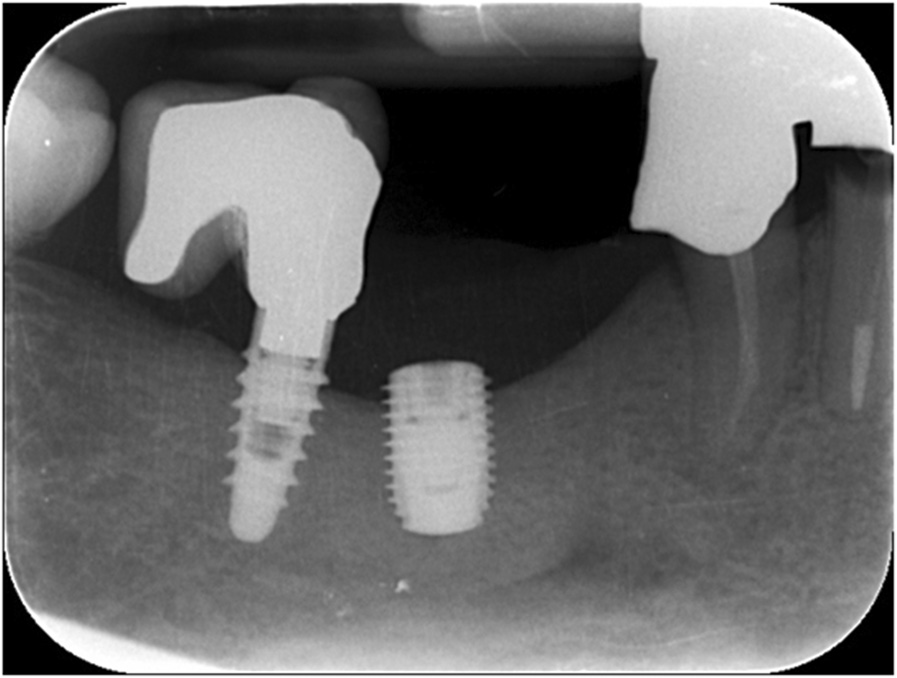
Intraoral radiography: implant placement
